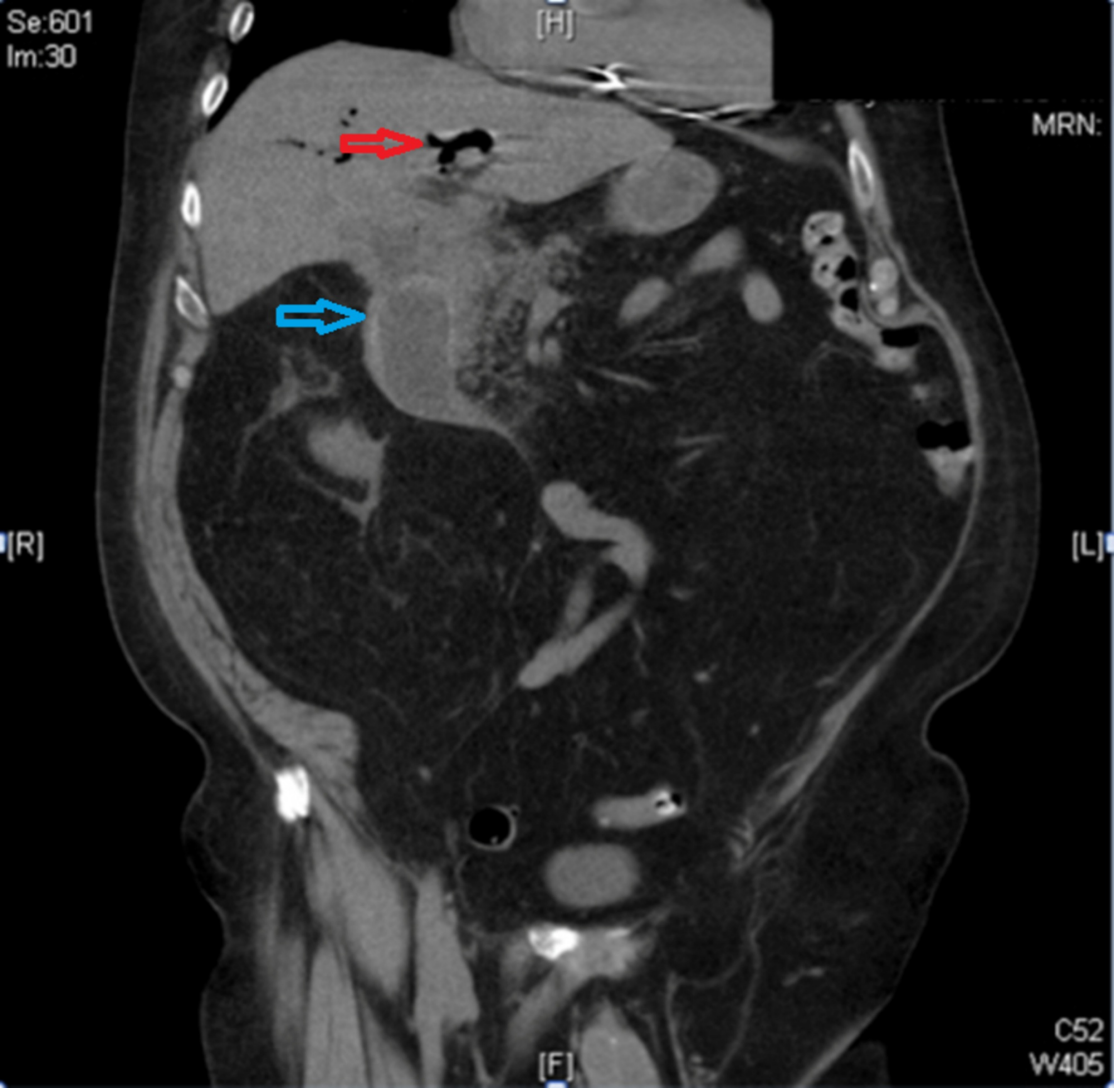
Computed tomography (CT) of the abdomen and pelvis showing impacted gallstone in the duodenum (blue arrow) and pneumobilia (red arrow)
